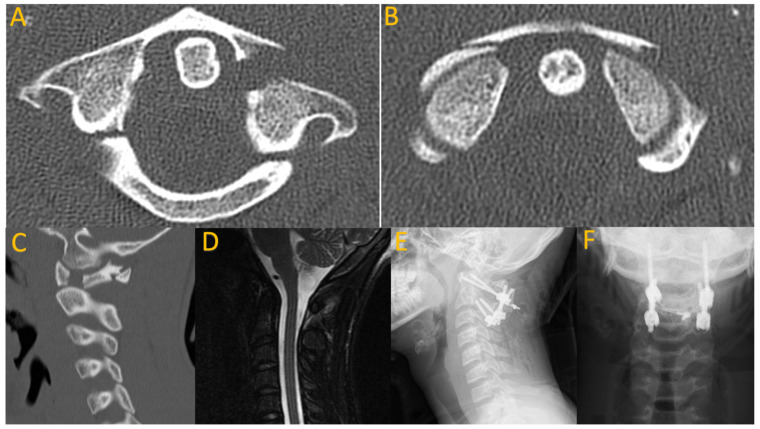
A 16-year-old male who presented with neck pain after diving into shallow water in the ocean, hitting a sand bar. He was neurologically intact. Axial (A,B) and sagittal (C) CT imaging reveals a four-part burst fracture of C1. Sagittal MRI STIR sequence suggests ligamentous injury (D). He underwent posterior C1–C2 fusion with instrumentation, wiring, and allograft (E,F).
